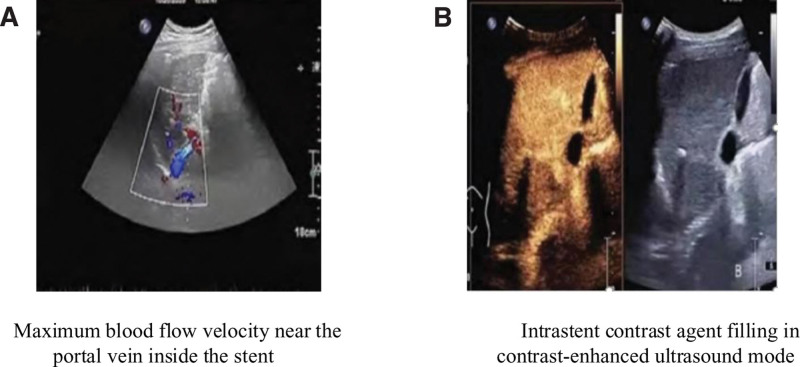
Color Doppler ultrasound and contrast-enhanced ultrasound examination.
